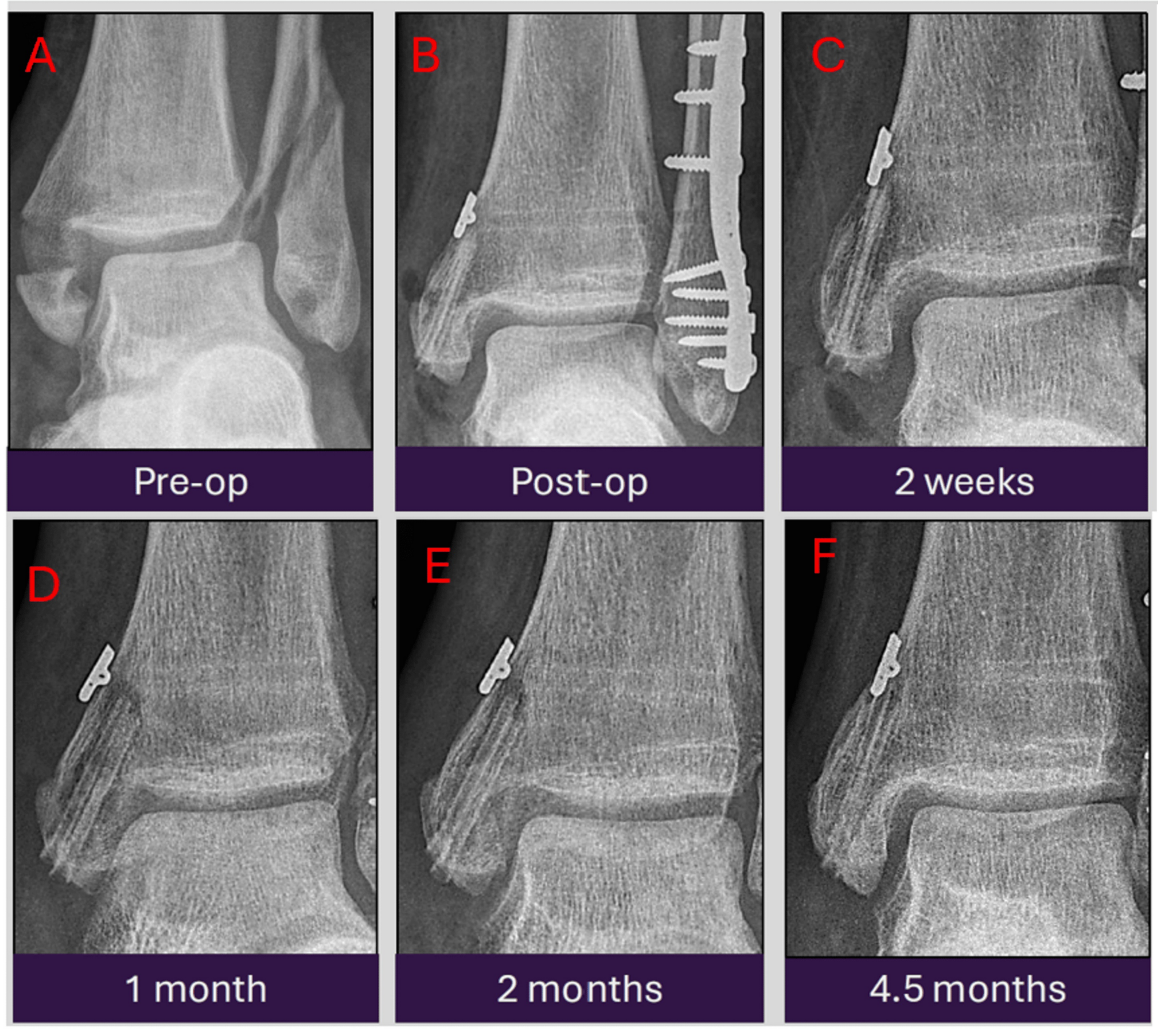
Serial antero-posterior radiographic images from pre-operation to 4.5 months post-operation showing persistent peri-implant radiolucencies which represent hydrogen gas released during implant biocorrosion, a benign and expected finding. The radiographs were obtained at pre-operation (A), immediately post-operation (B), two weeks (C), one month (D), two months (E), and 4.5 months (F) post-operation.
